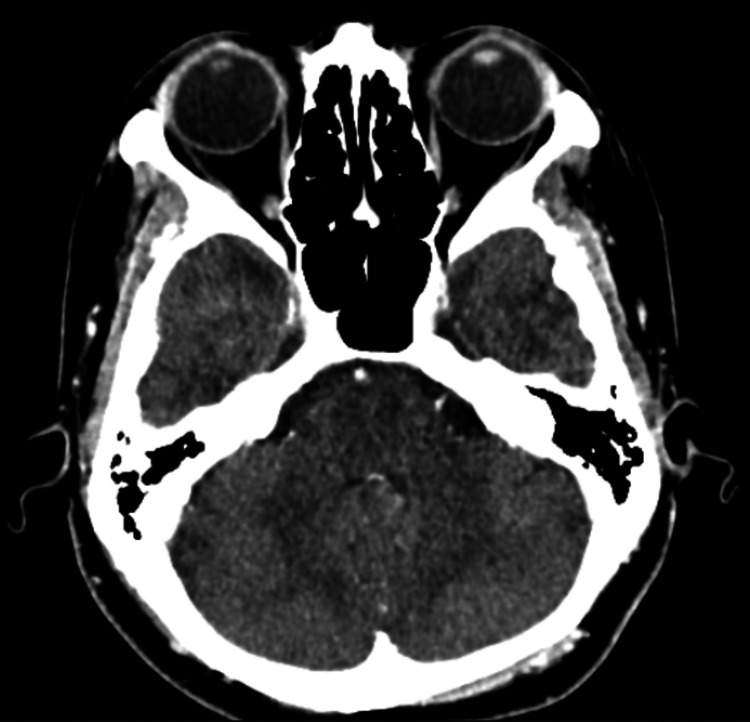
Contrasted CT of the brain revealed no focal or enhancing brain parenchymal lesion and no evidence of a space-occupying lesion.
